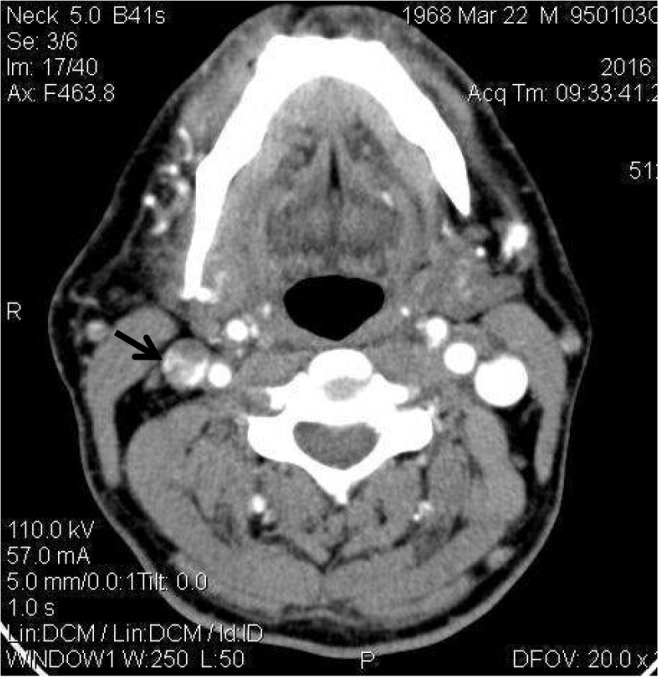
Axial veiw of the neck CT scan with IV contrast shows thrombosis in the right jugular vein (arrow)
